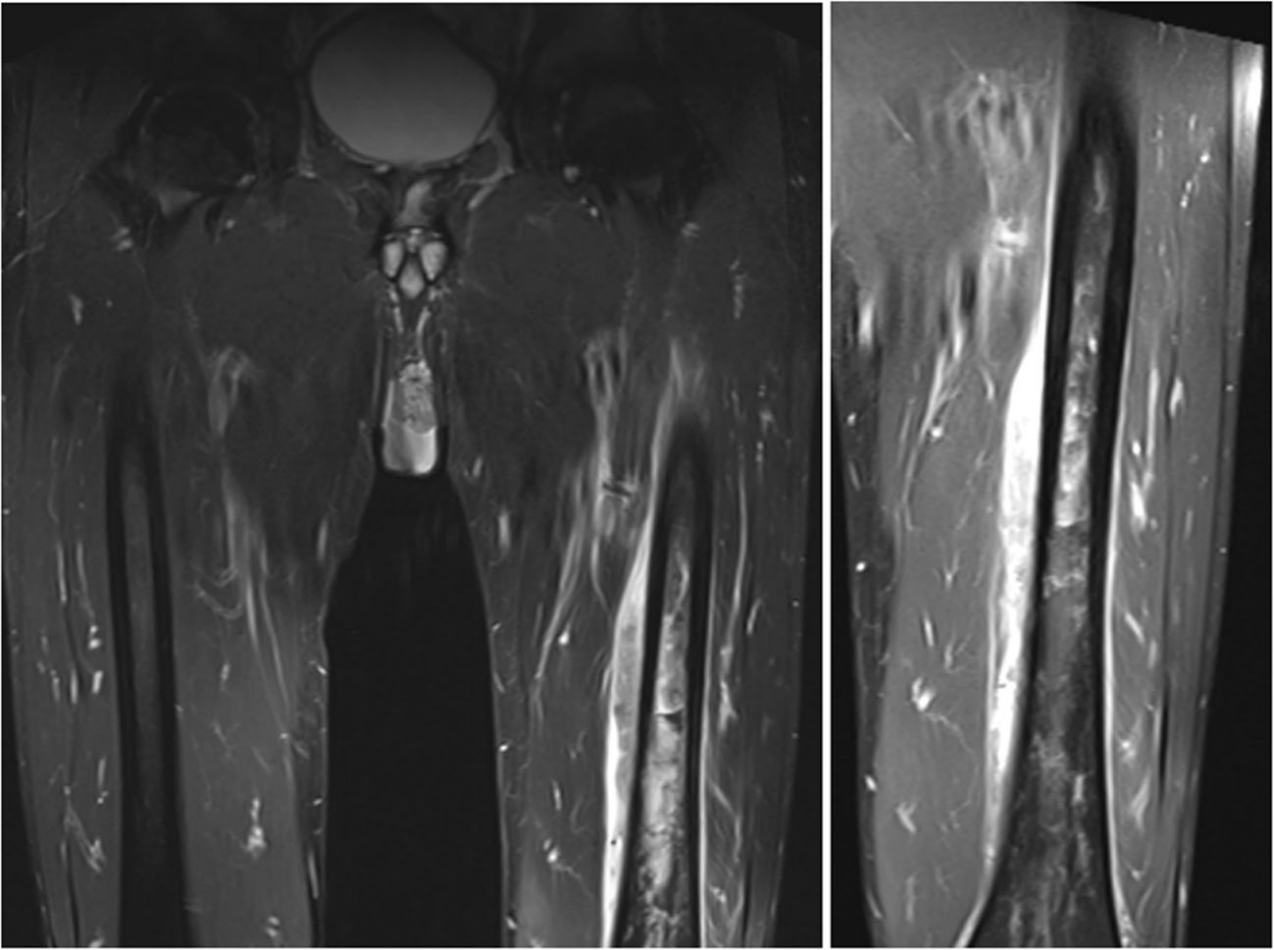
MRI scan (number 1) of both femurs (left) and a close-up of the left femur (right) showing a periosteal lesion in the left musculus vastus intermedius and a permeative process of the left femoral diaphysis
